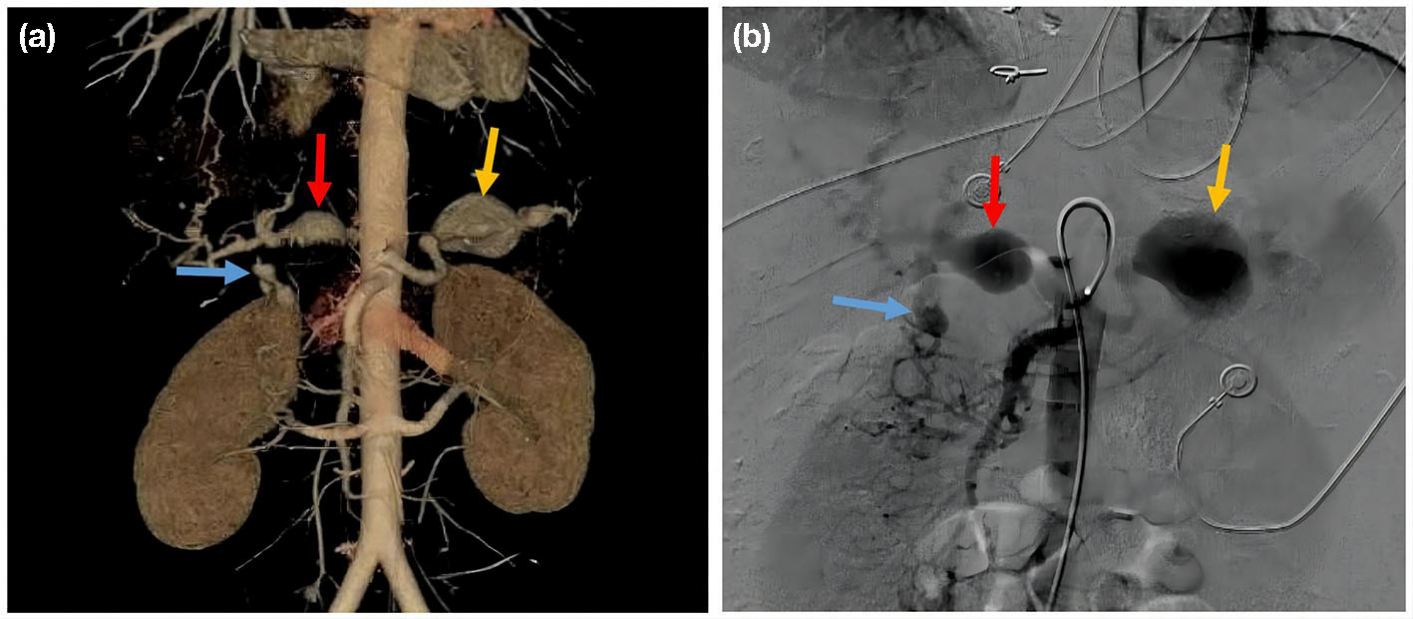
(a) Aortic CT angiography; splenic artery aneurysm (yellow arrow); common hepatic artery aneurysm (red arrow); gastroduodenal artery aneurysm (blue arrow). (b) Celiac artery angiography, splenic artery aneurysm (yellow arrow); common hepatic artery aneurysm (red arrow); gastroduodenal artery aneurysm (blue arrow).
